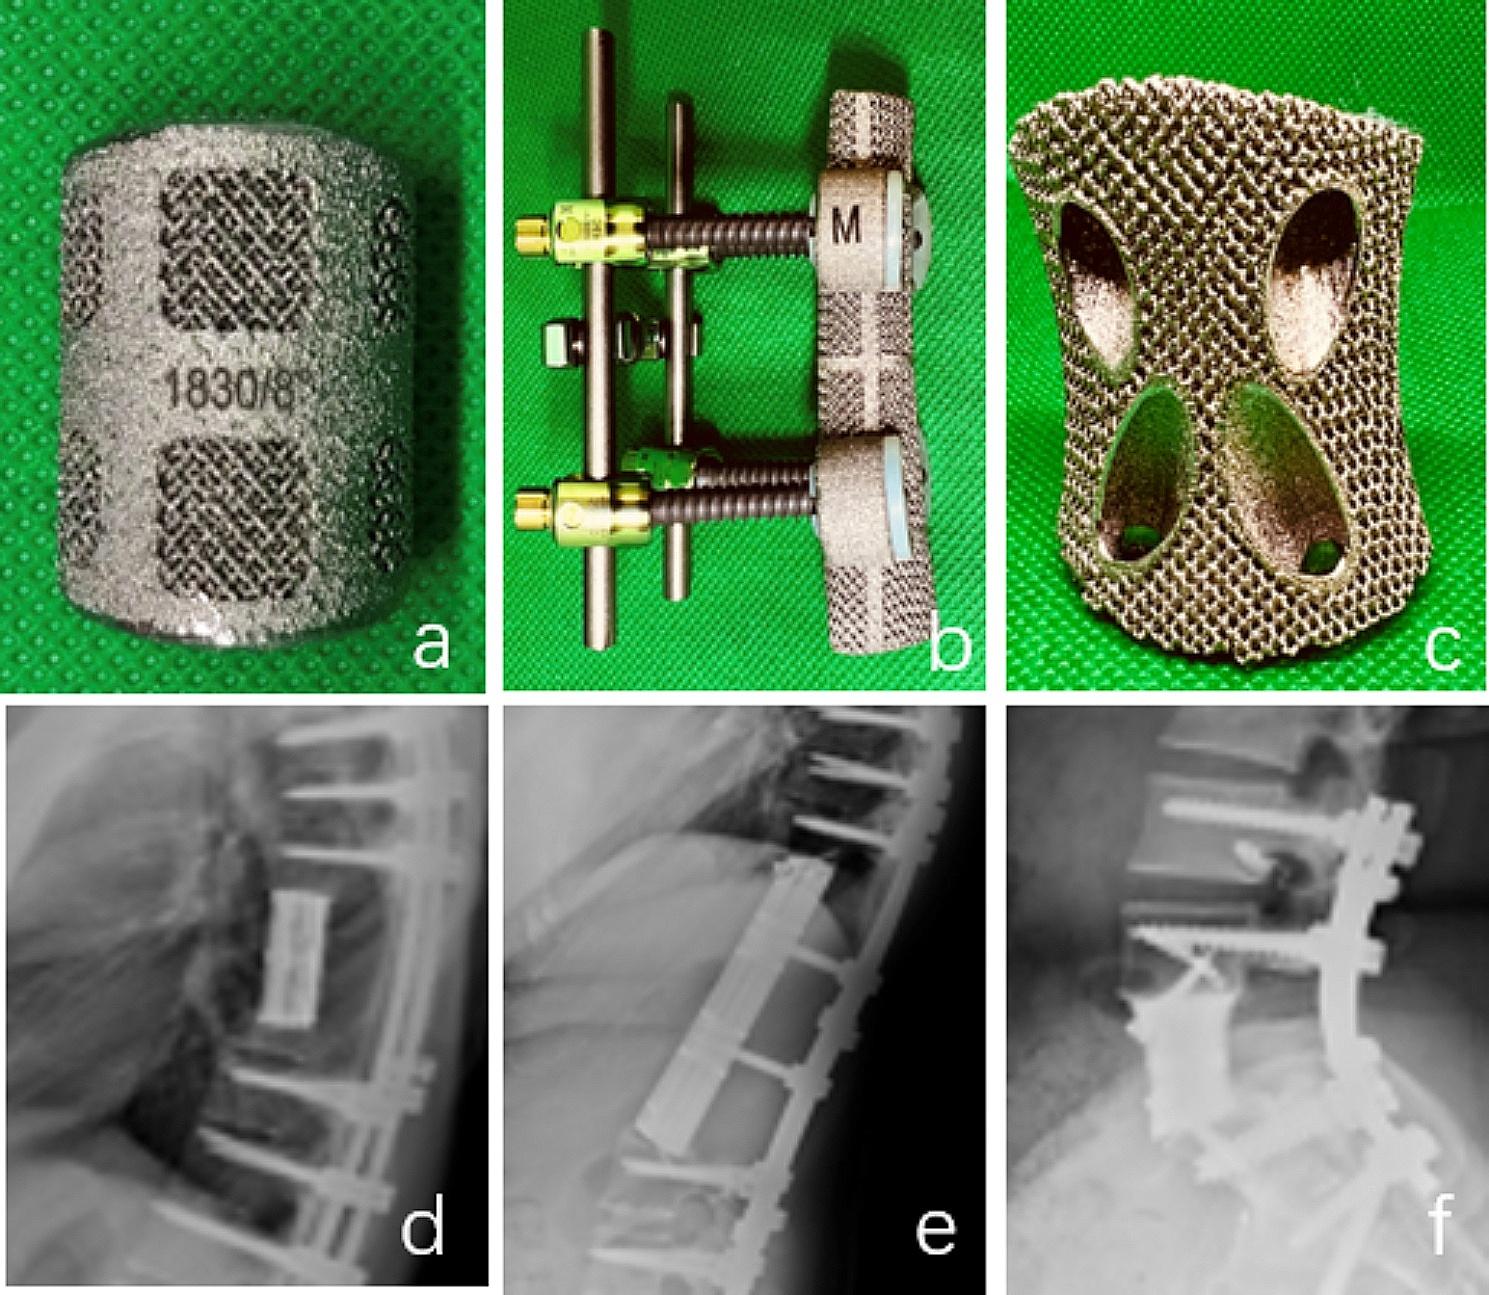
(a) Standard 3D-printed vertebral body. (b) Truss structure 3D-printed vertebral body. (c) Anterior self-stabilizing 3D-printed vertebral body-connection to vertebral body. (d) X-ray of standard 3D-printed vertebral body after surgery. (e) X-ray of truss structure 3D-printed vertebral body after surgery. (f) X-ray of Anterior self-stabilizing 3D-printed vertebral body after surgery
